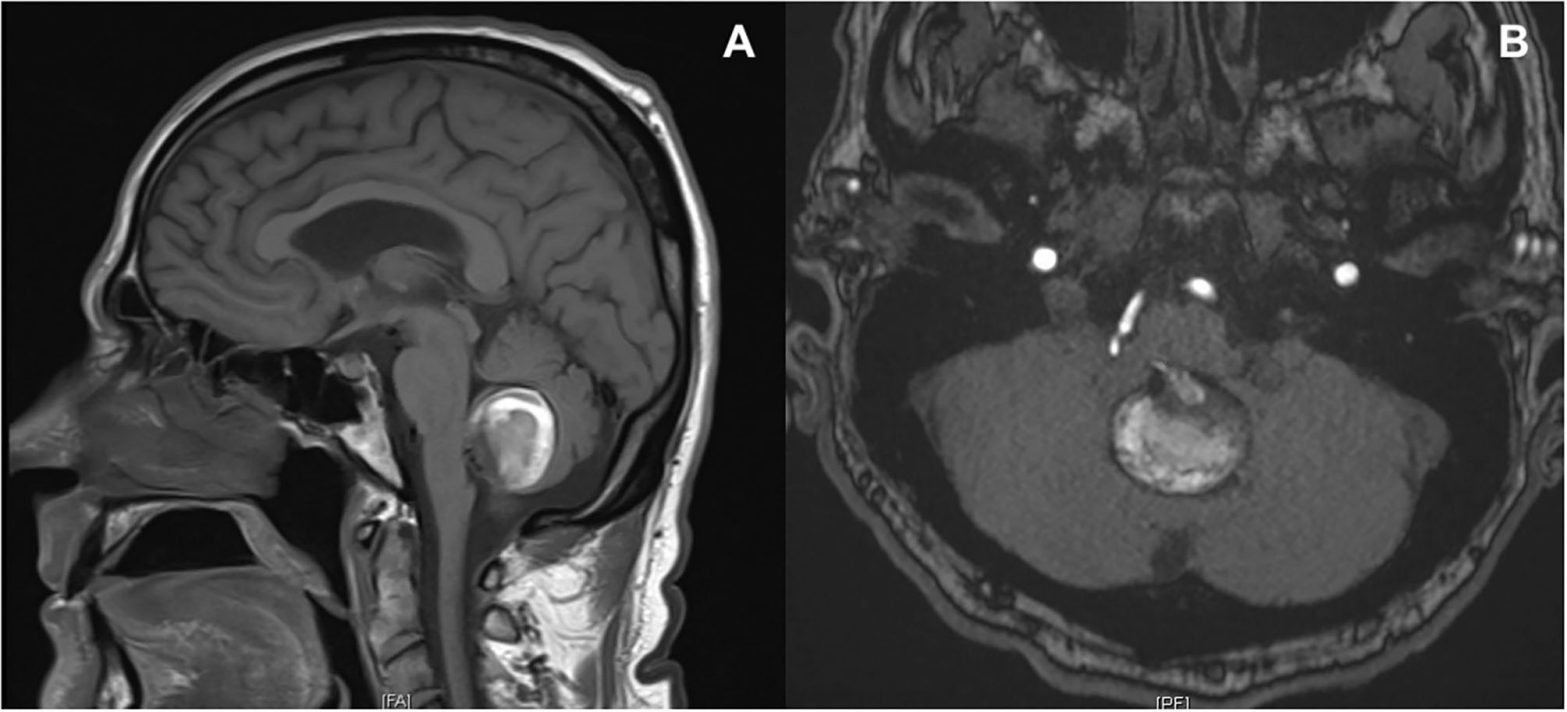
MRI T1 sequence (A) and time of flight acquisition (B). Centered within the midline of the posterior fossa, favored to lie just below the floor of the fourth ventricle, there is a 3.1 × 3.1 × 2.8 cm partially thrombosed aneurysm sac, which arises from the right PICA. There is a suspected patent lumen measuring ~8.5 × 5.5 mm. Aneurysm sac demonstrates a narrow neck, ~2 mm. There is no evidence of superficial siderosis or hemosiderin deposition.
